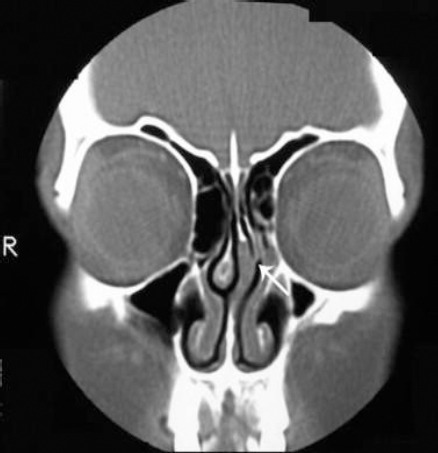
CT tomography of nasal fracture
